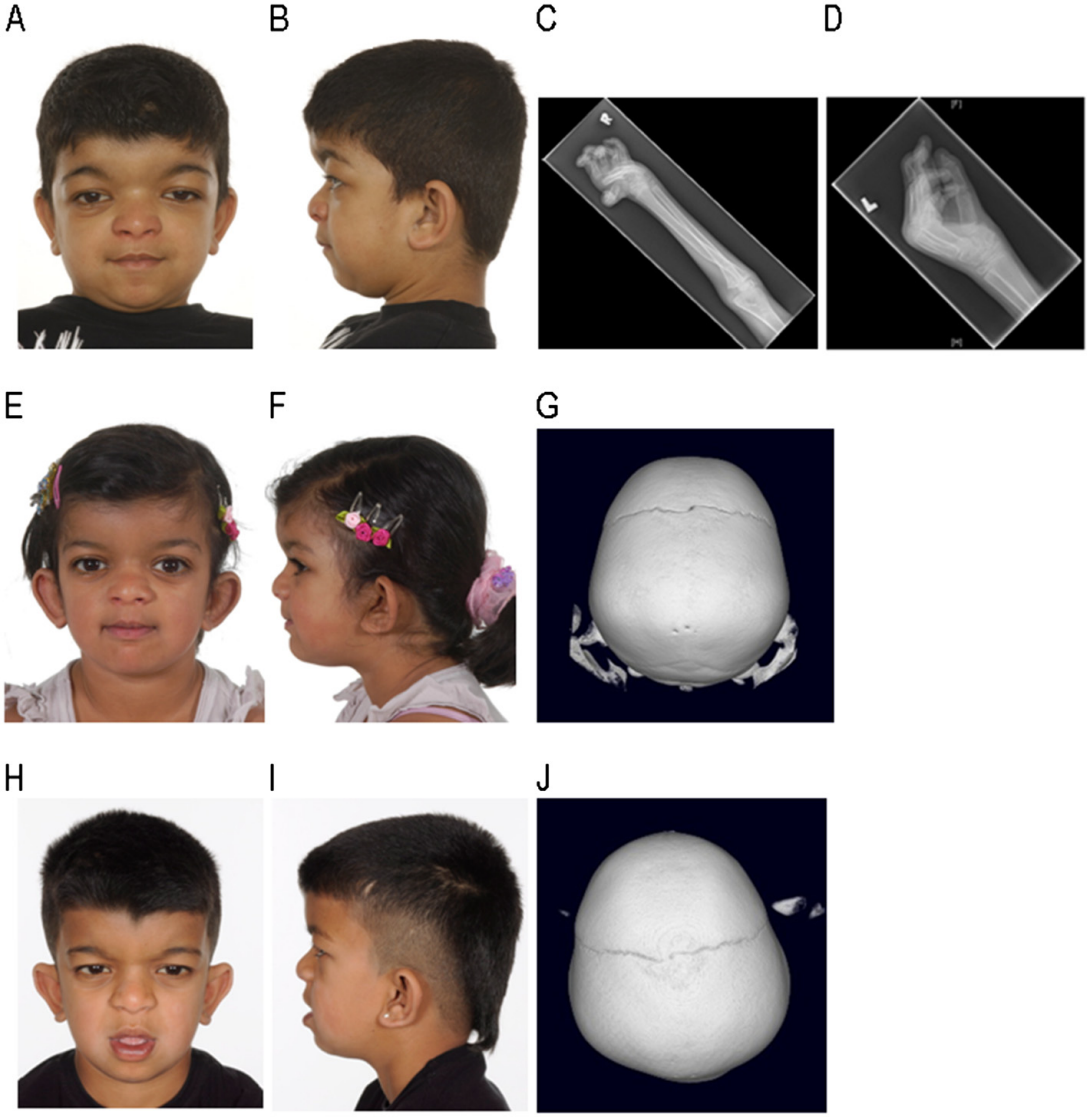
A-D: Patient 1.A: Antero-posterior (AP) view showing facial features including hypertelorism. B: Lateral view showing brachycephaly and micrognathia. C: X-ray (XR) right radius/ulna showing broad appearance of the radius at the junction between the proximal and middle thirds. D: XR left hand showing crowding of the carpal bones, broad metacarpals, proximal and middle phalanges, and flexion at the MCP and PIP joints. E-G: Patient 2.E: AP view showing facial features including hypertelorism. F: Lateral view showing brachycephaly. G: 3D CT scan showing absence of the sagittal suture. H-J: Patient 3.H: AP view showing facial features including hypertelorism. I: Lateral view showing class III malocclusion and brachycephaly. J: 3D CT scan showing absence of the sagittal suture.
